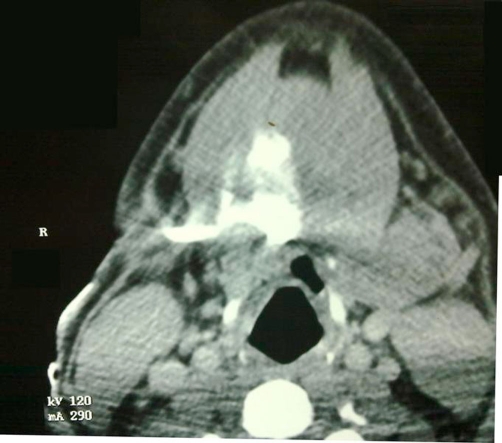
CT fistulogram showing the cavity below floor of mouth.
